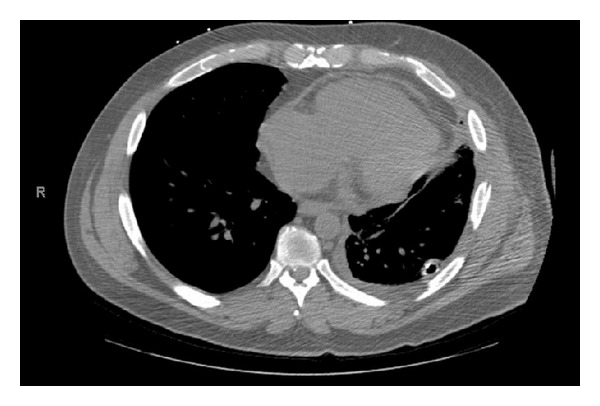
The CT study performed on postoperative day 7 that shows dramatic improvement in the cardiac position as well as decreased atelectasis of the lower lobe of the left lung.
